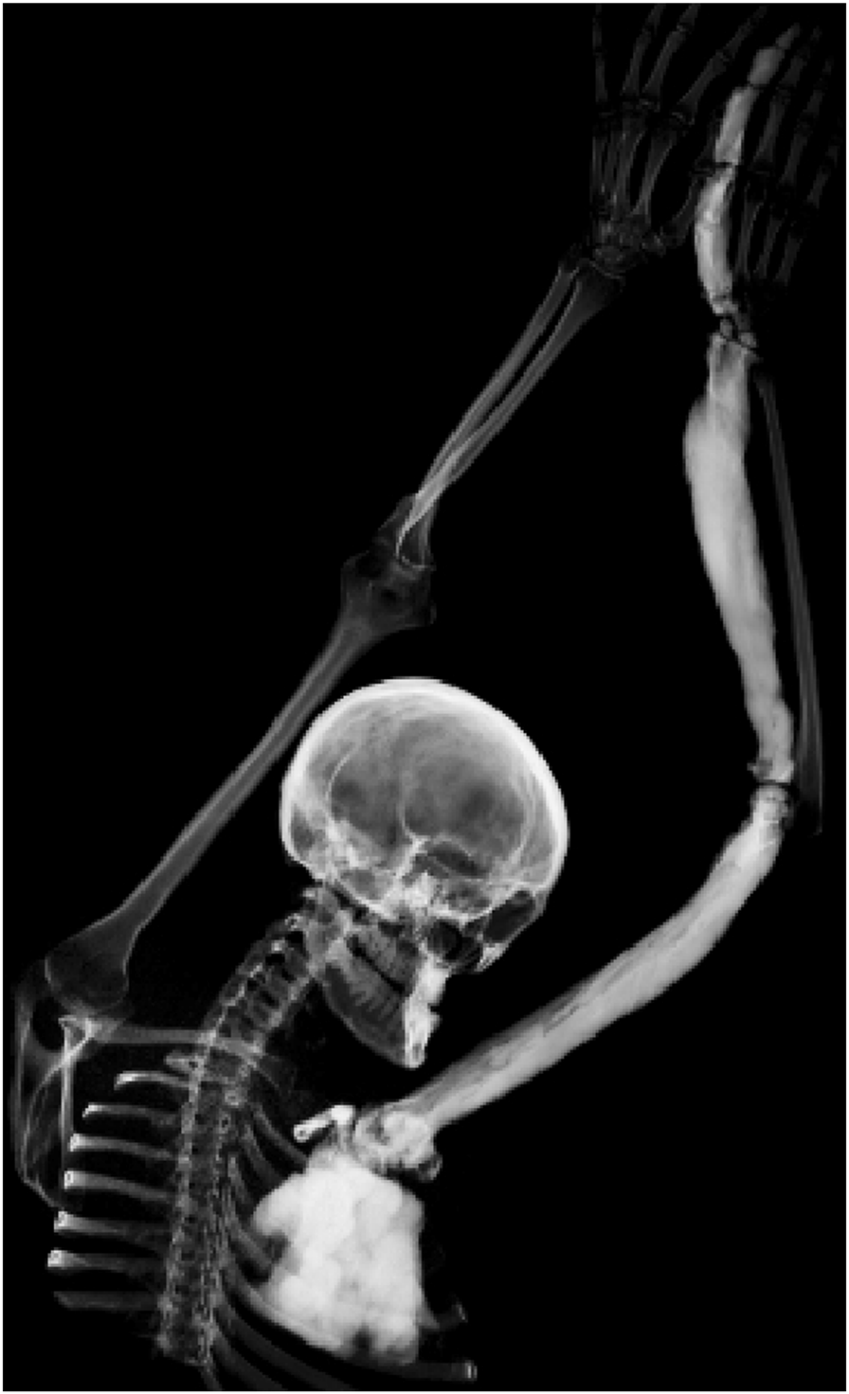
CT of left hand and forearm. Diffuse thickening and sclerotisation on index finger and II. metacarp shaft. The lesion is less aparent at the I. metacarp and on some carpal bones. There is diffusely enlarged sclerotic radius and humerus without ulna bridging. Whole scapula on the left side is also affected. The cavities on the affected bones are completely filled with the sclerotic bone.
